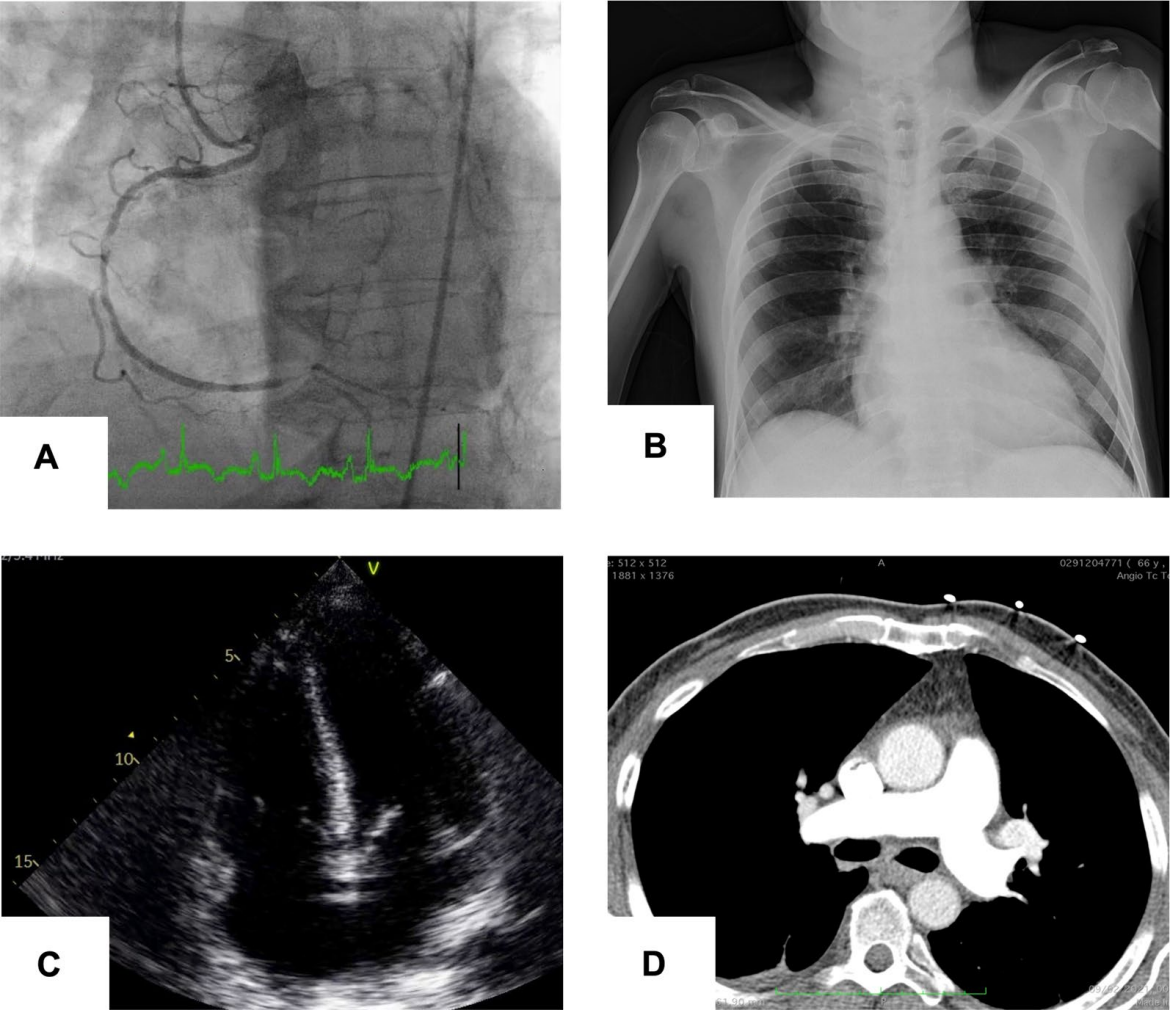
A Coronary angiography showing occlusion of the proximal right coronary artery. B Chest X-ray displaying absence of pulmonary congestion, consolidation or diffuse parenchymal infiltrate. C Transthoracic echocardiography on admission: apical four-chamber view showing right ventricular enlargement. D Computed tomography pulmonary angiogram excluding thrombus
